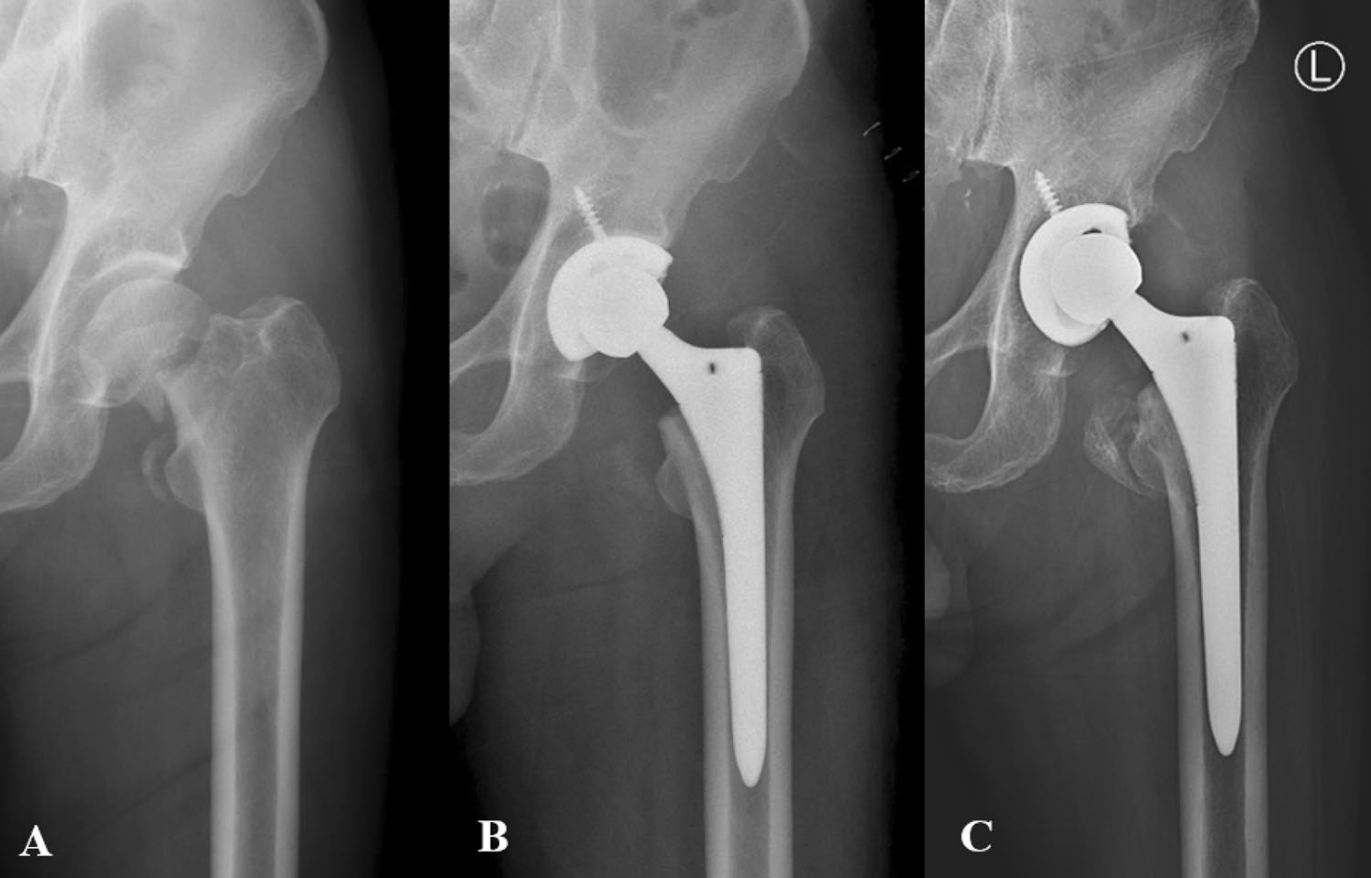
Serial radiographs of a 71-year-old patient who underwent total hip arthroplasty. (A) Left femoral neck fracture. (B) Elevated C-reactive protein level and wound complications on postoperative day 14. (C) A change in the acetabular cup angle at 7 months postoperatively.
